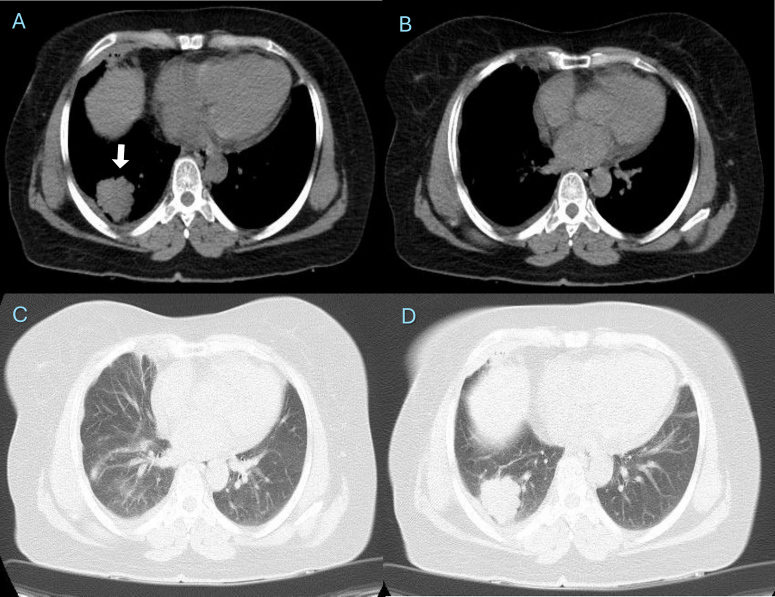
Axial CT images in the supine position performed 14 days post-biopsy showing near-complete resolution of the hemothorax and a stable right lower-lobe mass without recurrent fluid accumulation.
